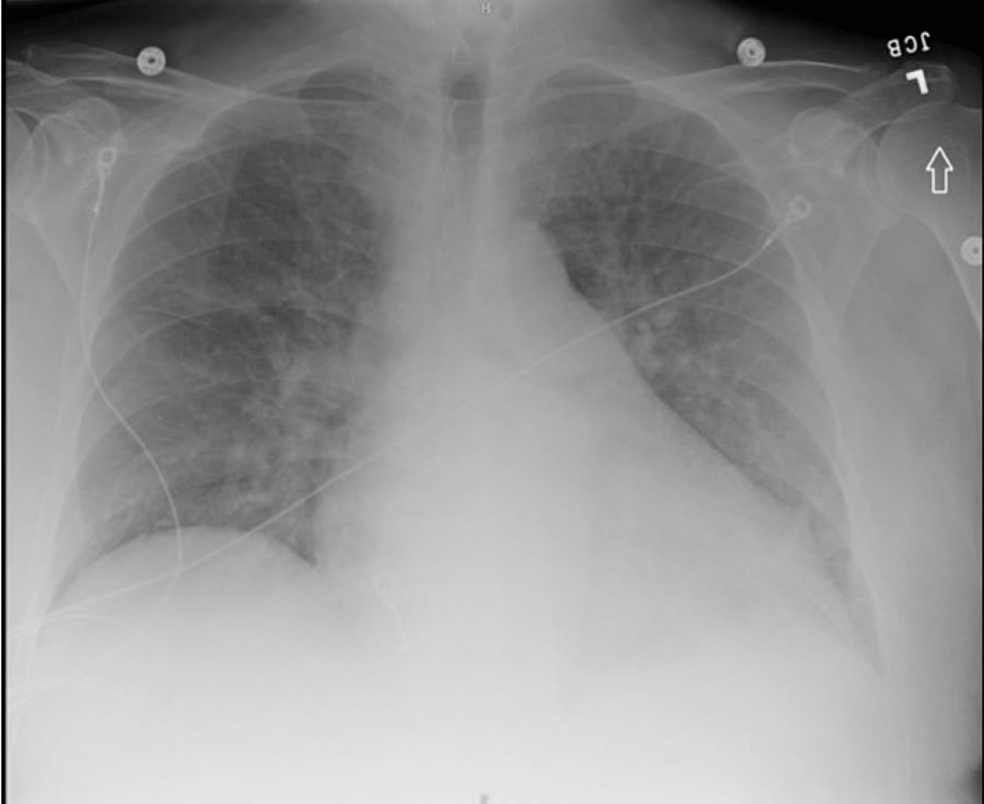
Chest radiograph showing cardiomegaly with pulmonary edema
